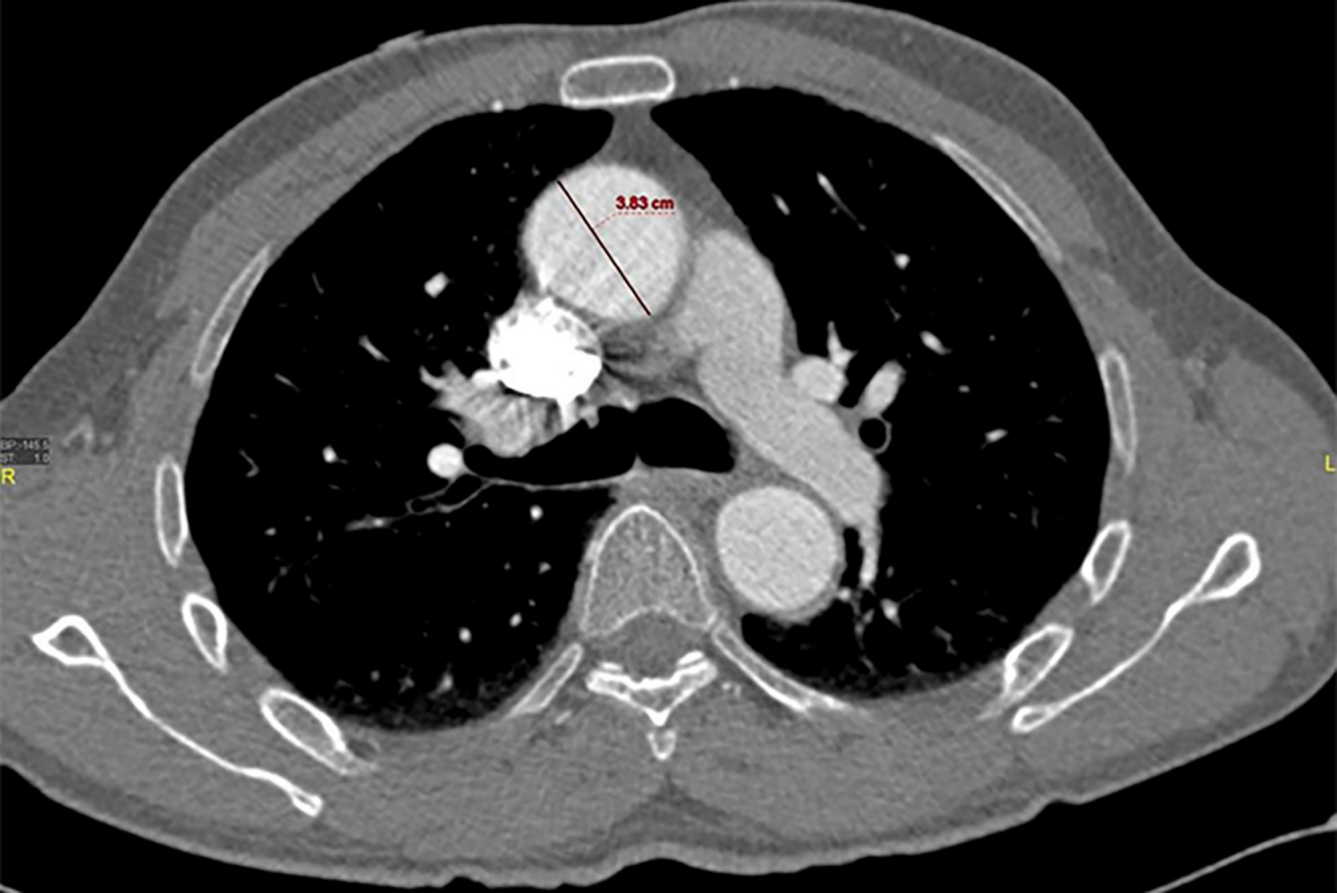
CT aortogram (axial image) demonstrating ectatic ascending aorta.
